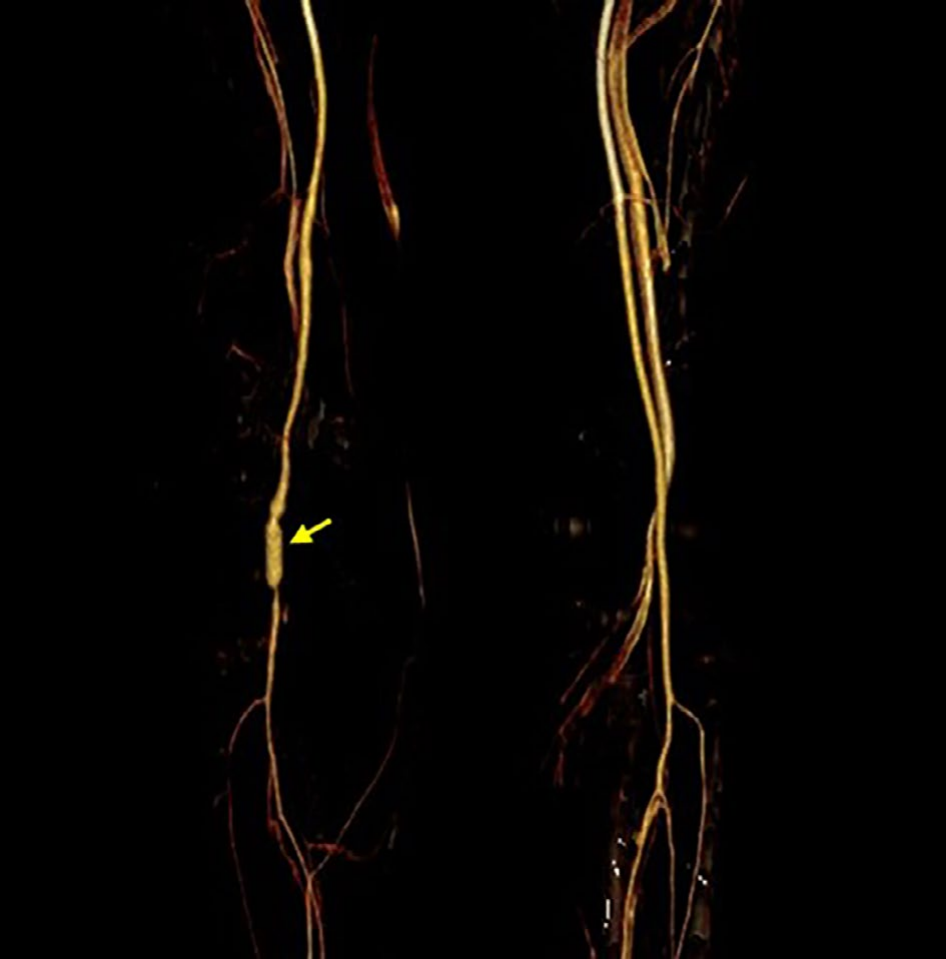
Postoperative CTA showed the patency of blood flow at the site of the great saphenous vein transplantation (yellow arrow).
